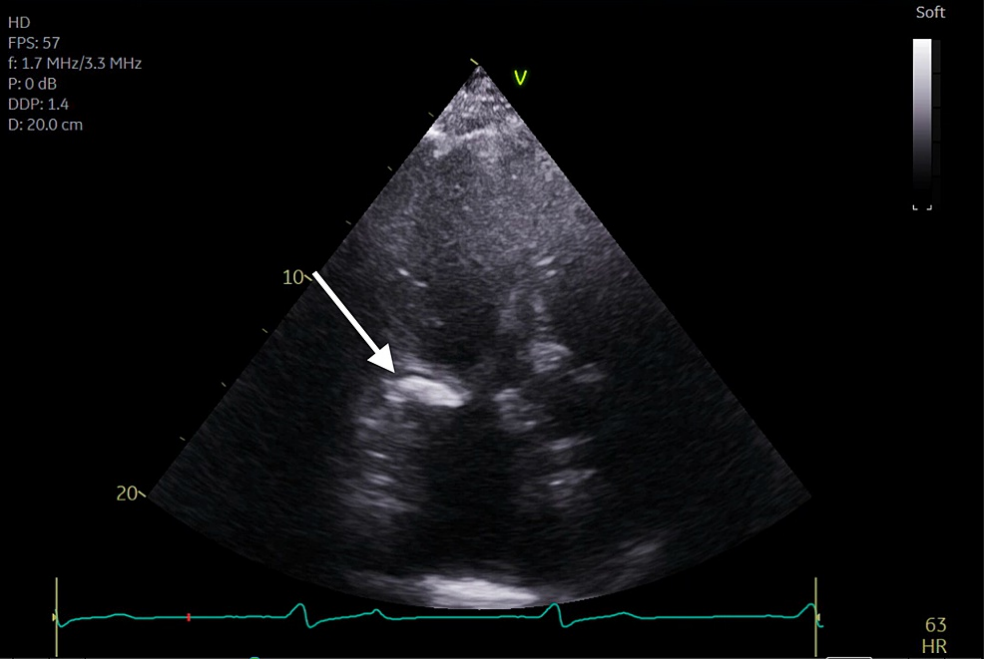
Transthoracic echocardiogram apical two-chamber view demonstrating severe mitral annular calcification.
